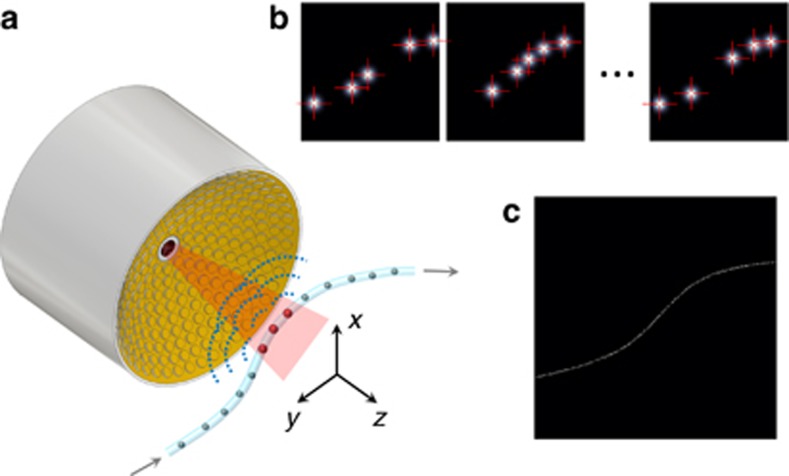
Imaging principle of localization optoacoustic tomography. (a) A spherical array of ultrasound transducers is used to acquire a three-dimensional optoacoustic image of flowing absorbers for each laser pulse. (b) The positions of sparsely distributed absorbers are measured (localized) in a sequence of images. (c) An image is formed by superimposing the localized positions.
